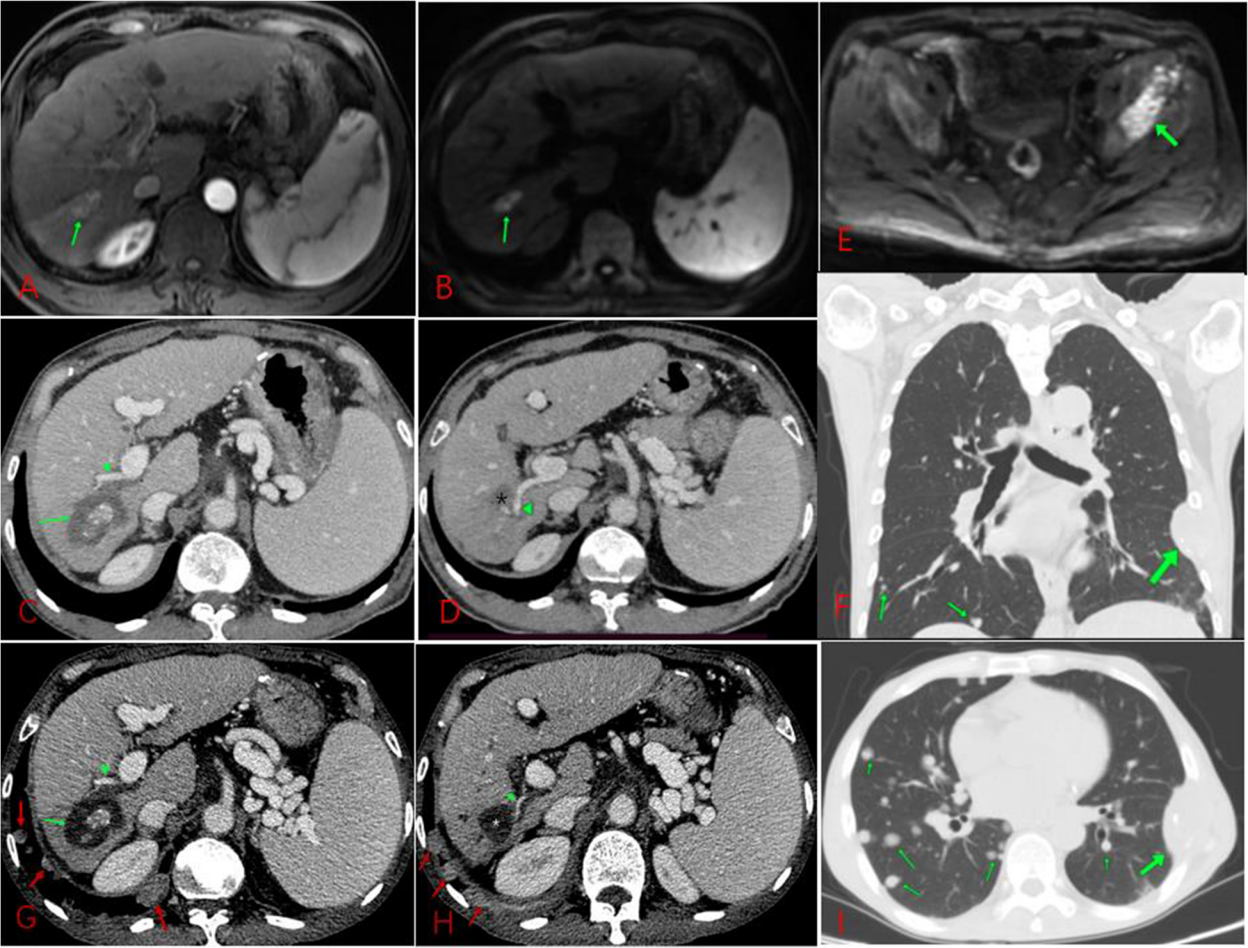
Extrahepatic recurrence with rapid progression after TACE+RFA for periportal HCC in a 61-year-old man. (A-B) DCE-MRI: hepatic arterial phase (A) and DWI (B) showing a small HCC (arrow) in the periportal location before TACE+RFA. (C-D) CT scan obtained during the portal venous phase 3 months after TACE+RFA showing the complete ablation zone (arrow) adjacent to the portal vein (arrowhead). (E) DWI scan obtained 6 months after TACE+RFA shows bone metastasis (arrow). (F) CT scan obtained 6 months after TACE+RFA showing thoracic wall metastases and multiple lung metastases (arrow). (G-I) CT scan obtained during the portal venous phase 12 months after TACE+RFA showing the complete ablation zone (arrow) adjacent to the portal vein accompanied by thoracic wall and lung metastases
